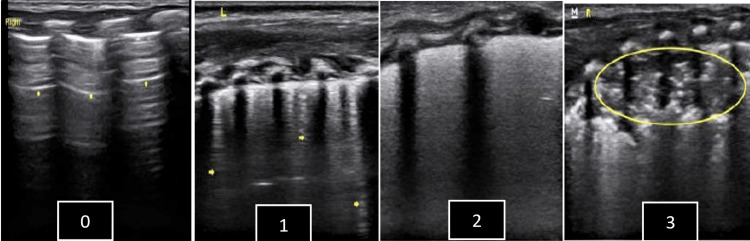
Description of the lung ultrasonography score. Scores are given as follows for any lung area: 0 indicates A-pattern (defined by the presence of only A-lines, arrow); 1 indicates B-pattern (defined as the presence of ≥ three B-lines, arrow, well-spaced); 2 indicates severe B-pattern (defined as the presence of crowded and coalescent B lines with or without consolidations limited to subpleural space); and 3 indicates extended consolidation (circle).
